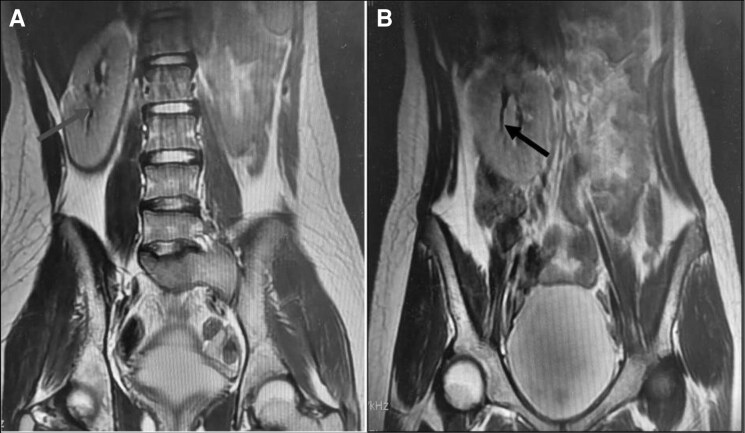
Coronal section of magnetic resonance image of the abdomen in case 4 described in Table 1, showing crossed-fused renal ectopia on the right side with (A) one kidney with the pelvis oriented outward (grey arrow) and (B) the second kidney with pelvis oriented inward (black arrow) and fused with the other kidney.
